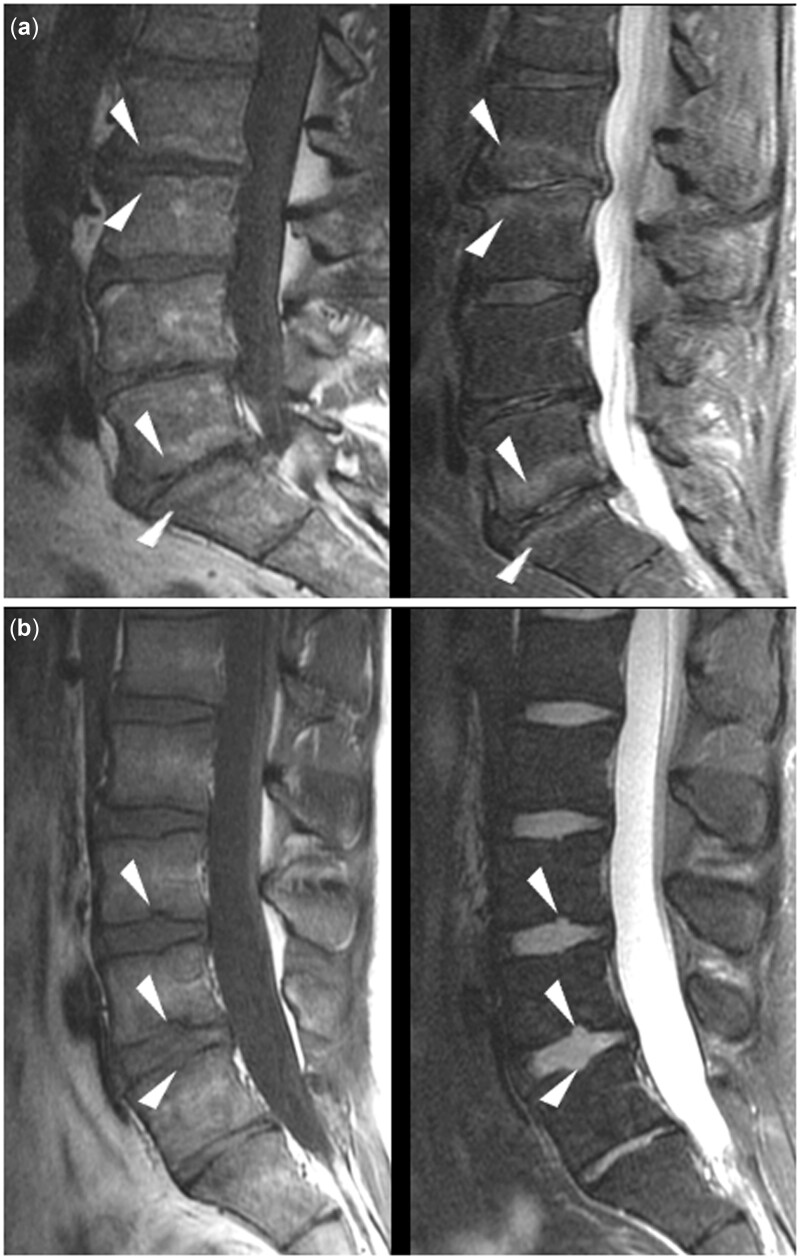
Endplate defects. Examples of endplate defects with degenerative appearance (erosive osteochondrosis) on T1- and T2-weighted sequences (a; arrowheads) and focal Schmorl’s nodes on T1- and T2-weighted sequences (b; arrowheads).
